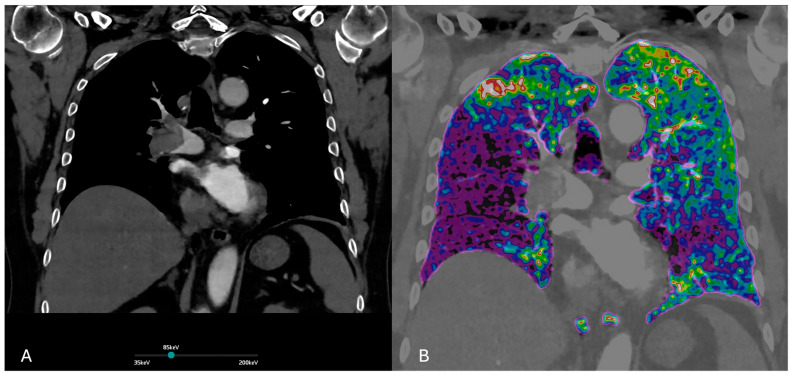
(A) Dual-energy CT. Virtual monochromatic at 85 keV image enhances vascular contrast while minimizing noise and beam-hardening artifacts. This reconstruction optimizes visualization of pulmonary embolism within the pulmonary arteries. (B) Iodine perfusion map demonstrates the regional lung perfusion. Areas of reduced iodine uptake (shown in purple) correspond to perfusion defects due to embolic obstruction of the pulmonary arteries.
